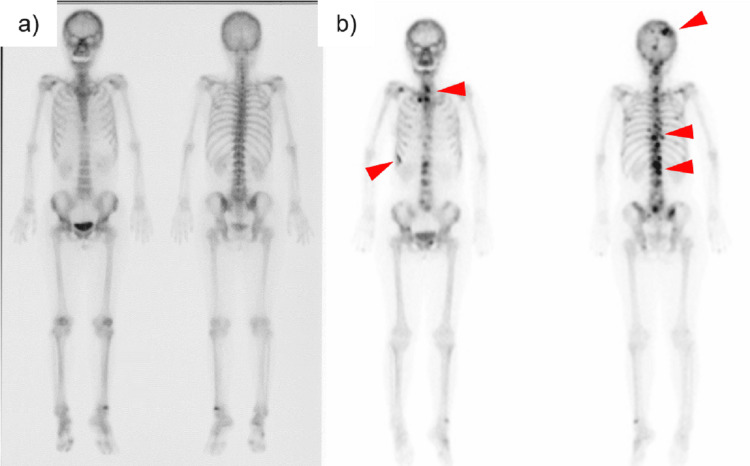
Bone scintigraphy showing no abnormalities in bone metabolism at the time of the initial diagnosis (a). After two courses of chemotherapy, it shows increased uptake in vertebrae, ribs, pelvic bone, and cranial bone (red arrowheads) (b).
